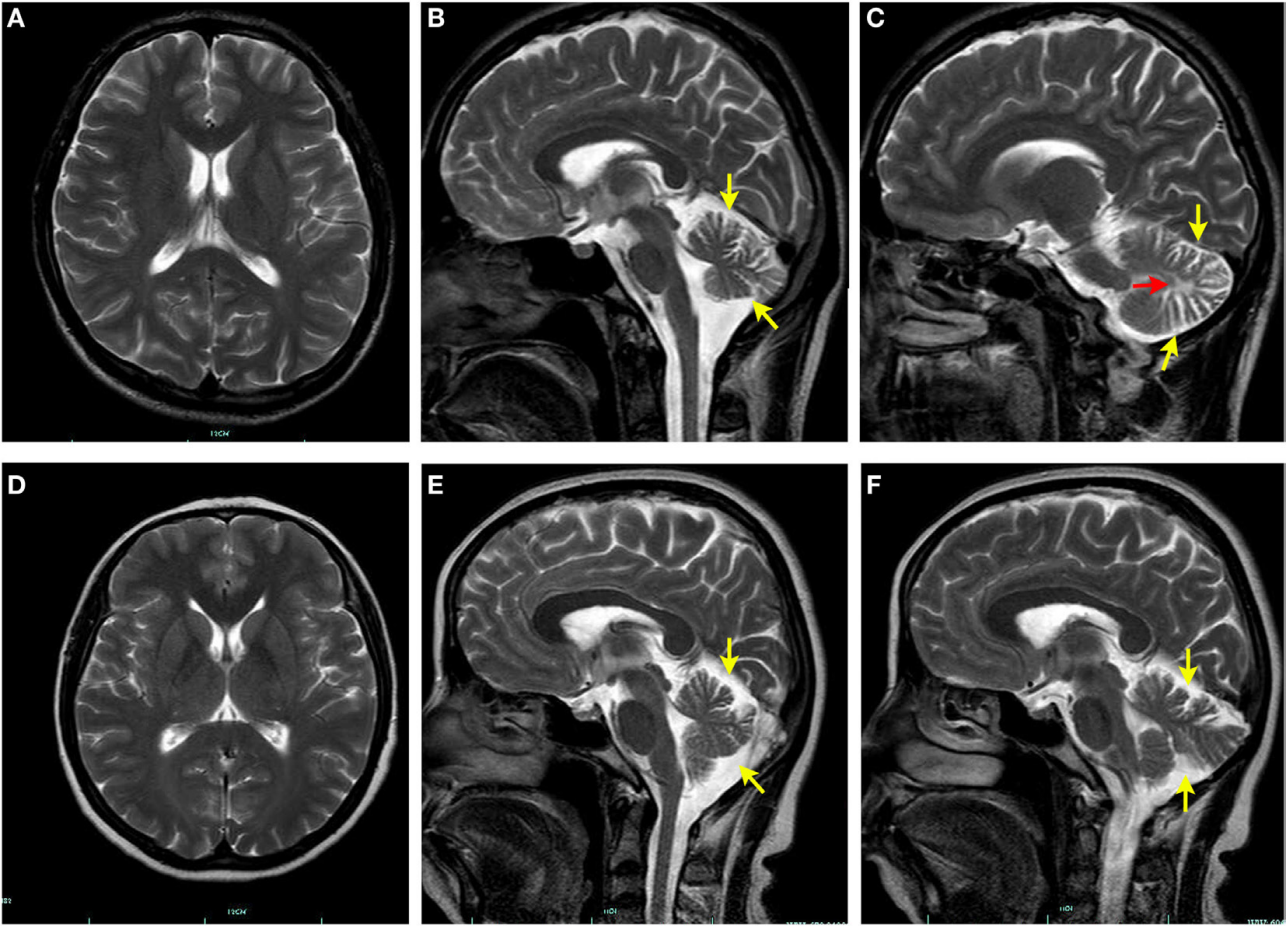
Brain magnetic resonance imaging (MRI) findings. No signal changes are seen in the axial T2-weighed brain MRI of the proband (A) and his mother (D). Sagittal T2-weighed brain MRI of the proband (B,C) and his mother (E,F) shows manifest cerebellar atrophy (yellow arrow). White matter hyperintensity (red arrow) is also evident in cerebellum of the proband (C).
